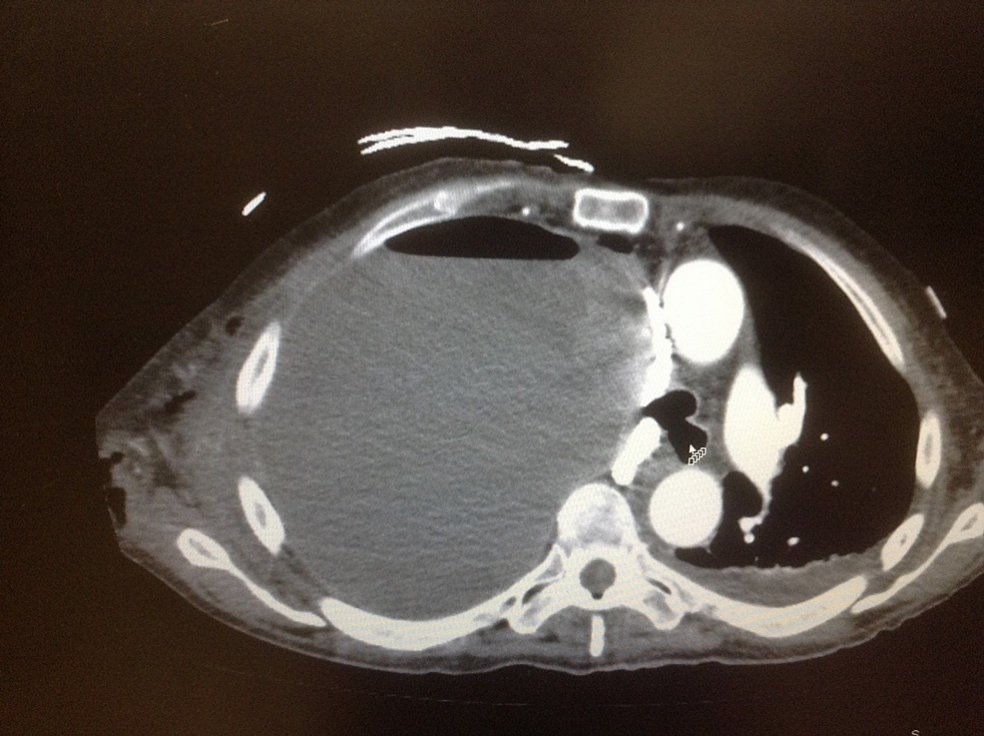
CT scan of the chest cavity. The arrow indicates one of the air-fluid level in the empyema necessitans. CT: computerized tomography
